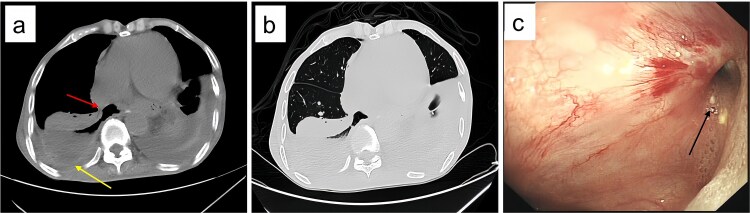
Post-admission chest CT and gastroscopy. (a) Mediastinal window showing esophageal fistula (arrow) and empyema (arrow); (b) lung window confirming the 67 × 36 × 117 mm right empyema; (c) gastroscopy identifying a 8 mm fistula (arrow) 38 cm from incisors.
